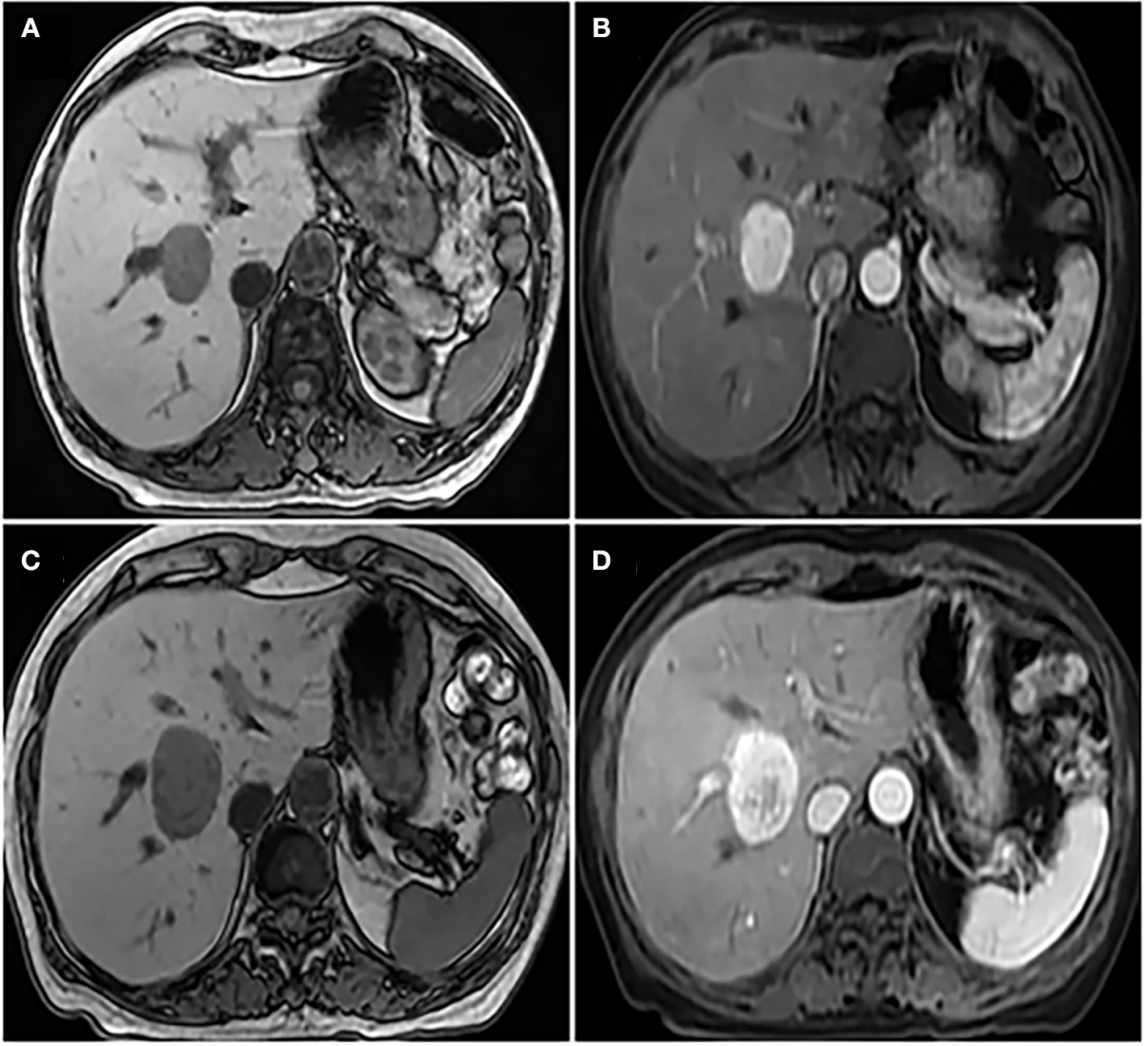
(A) Non-enhanced abdominal MRI showed that there was an occupying mass in the right lobe of liver, the boundary of tumor was unclear; (B) enhanced abdominal MRI indicated mild heterogeneous enhancement of solid components around the tumor and the size of the tumor was ~2.7 × 2.8 × 3 cm; (C) a second non-enhanced abdominal MRI showed that the mass in the right lobe of liver grew larger, and the size was ~3 × 3.5 × 3.5 cm; (D) a second enhanced abdominal MRI showed the low density of the cyst was seen in the tumor, which was considered to be accompanied by hemorrhage.
